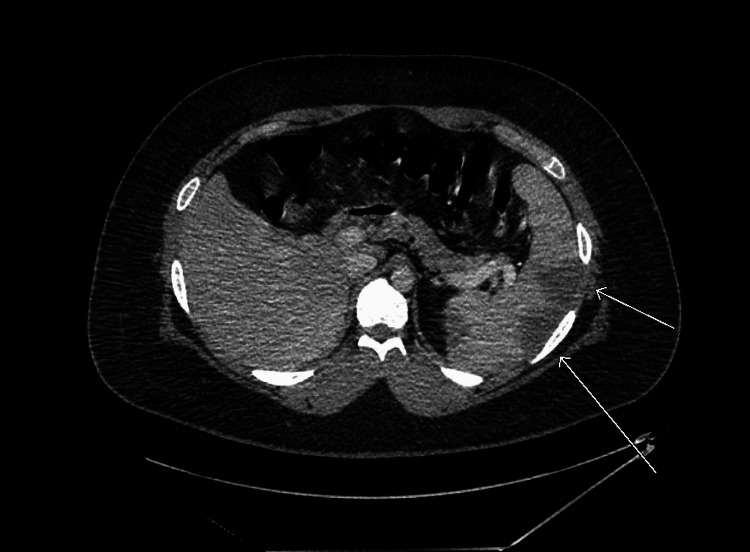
Axial CT image demonstrating multiple splenic infarcts (white arrows).
